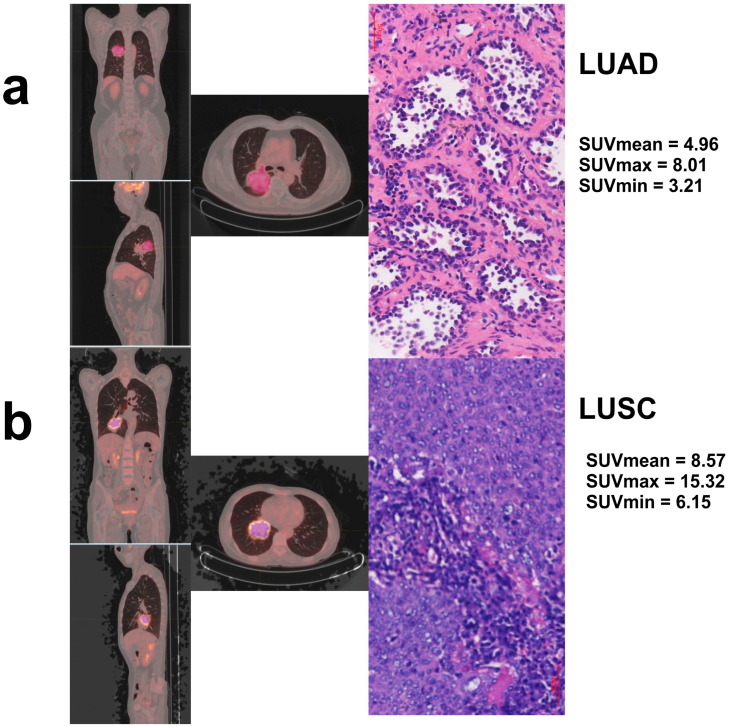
Typical cases of LUAD and LUSC. PET/CT image of a 68-year-old woman, the total score in the nomogram was 201.453, the probability of LUAD was 0.72, and the postoperative pathology confirmed LUAD (a). PET/CT image of a 54-year-old man, the total score in the nomogram was 53.902 and the probability of LUSC was 0.81, and the postoperative pathology confirmed LUSC (b).
